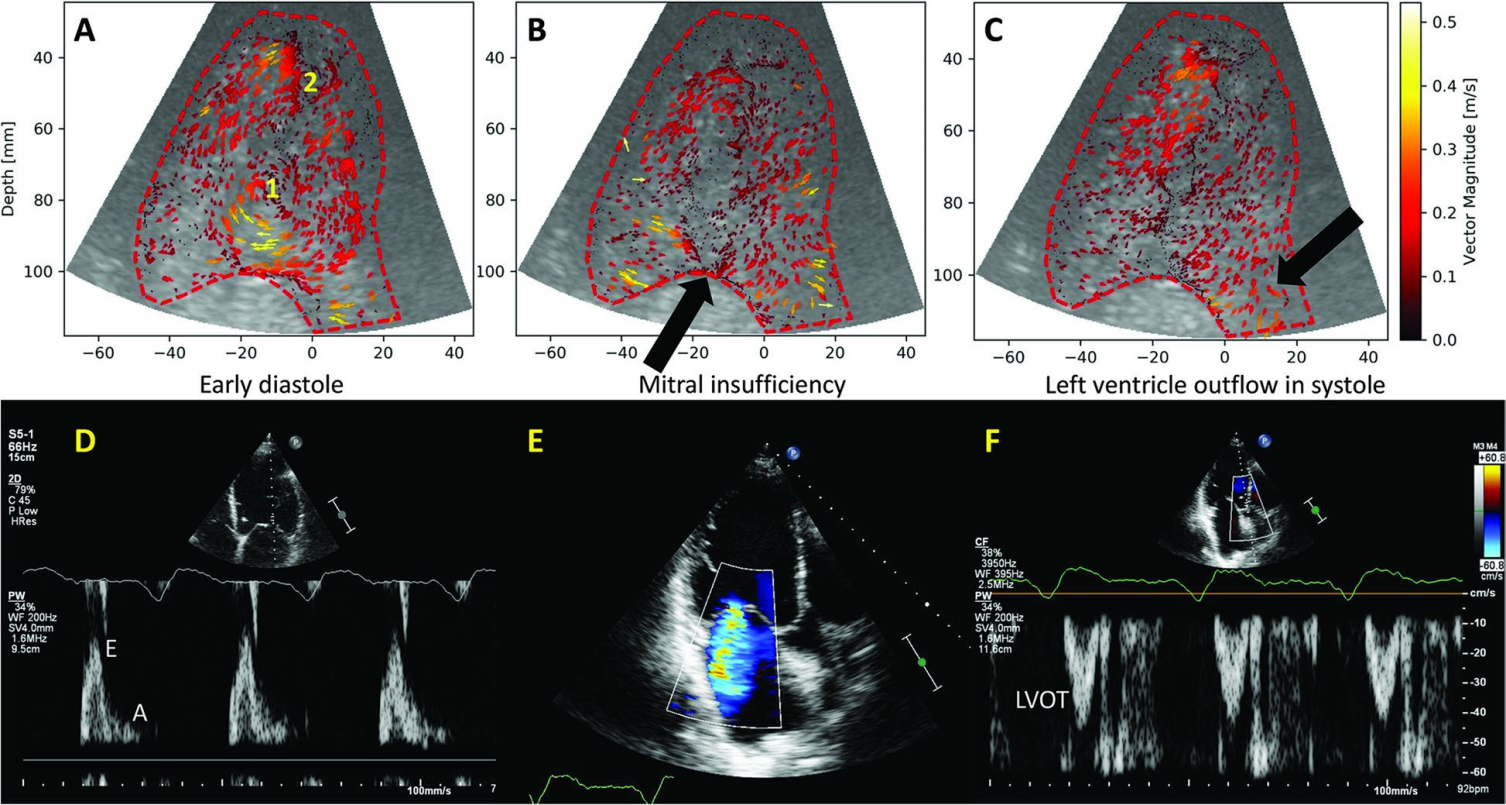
Patient 3. A: There were two main diastolic vortices (1,2), triggered by the restrictive mitral inflow and intraventricular recirculation of flow. Both vortices rotated clockwise, generating a conflicting flow at the interface between them, with mid-LV stasis and increased recirculation in the apex (Additional file 11). (D); B: In systole, a part of the downwards-going flow converged into the mitral insufficiency jet, while the outflow tract displayed low-velocity flow (black arrow; E color Doppler); C: low-velocity LVOT flow (black arrow); F: LVOT PW Doppler confirms the low flow
